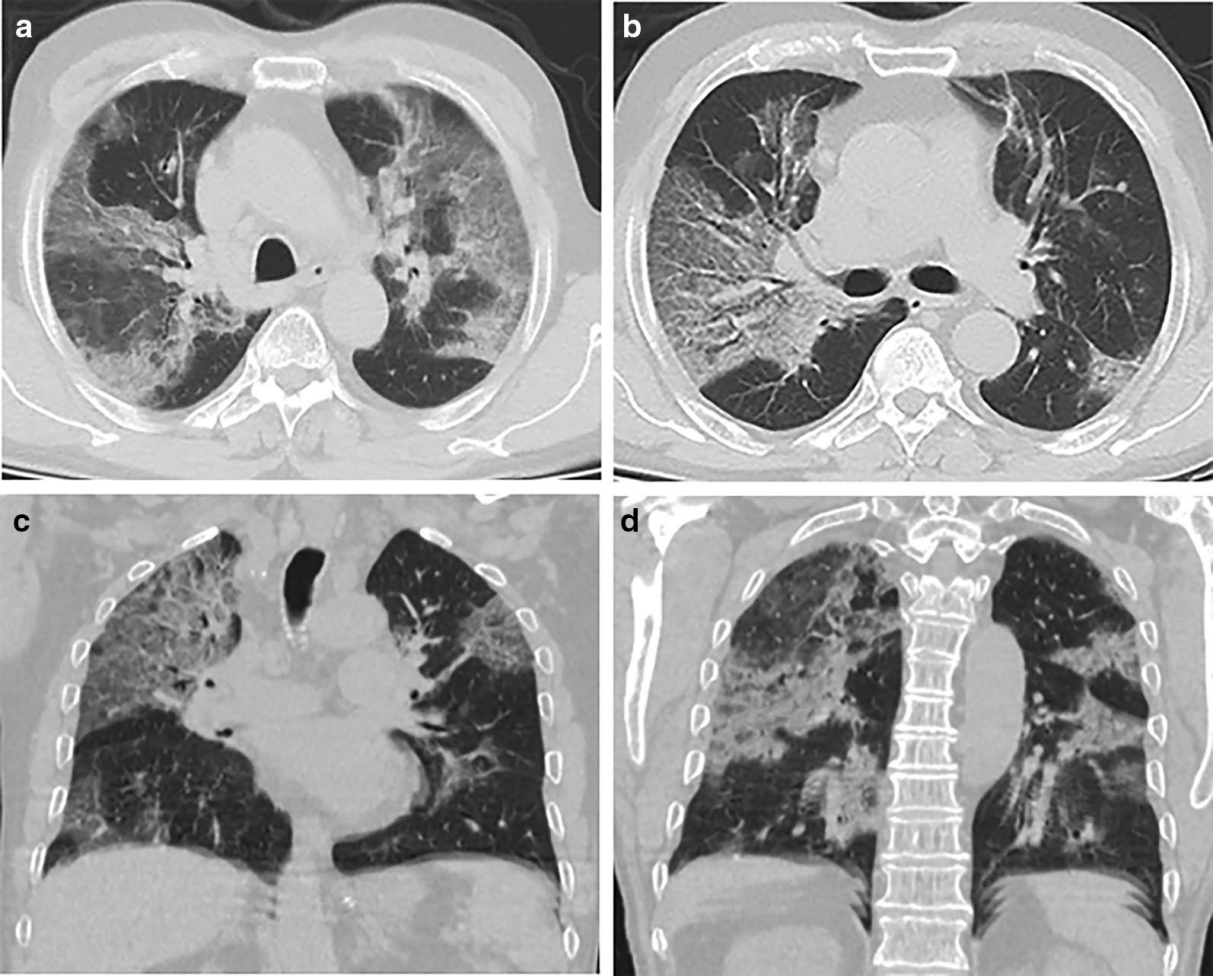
A 67-year-old man with positive RT-PCR findings for COVID-19. a–d Noncontrast axial CT images of the chest show bilateral, multifocal peripheral ground-glass opacities with superimposed interlobular septal thickening and intralobular lines are visible, giving the appearance of “crazy-paving”. This is in keeping with his CO-RADS 5 and typical RSNA classification
